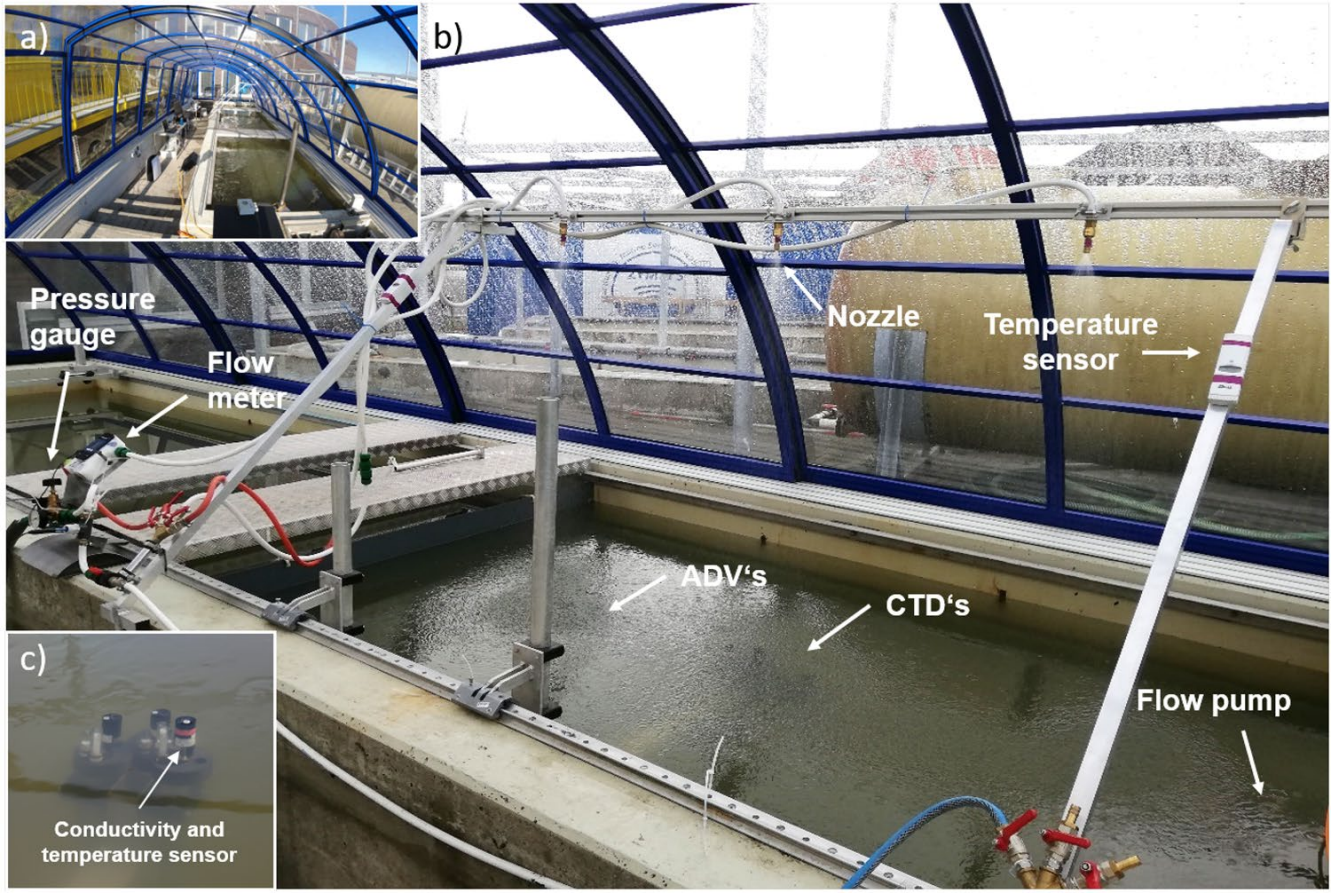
(a) SURF facility with the entire length of the covered tank and (b) tank of SURF with the experimental set up, including the artificial rainfall unit with the pressure gauge and flow meter to regulate the rain intensity, the acoustic doppler velocimeter (ADV), and the conductivity temperature depth sensors (CTD) placed inside the tank on a position below the nozzle. The positions of the flow pump and temperature sensors are also shown. (c) The CTDs in a vertical upward position with the conductivity and temperature sensor close to the surface.
